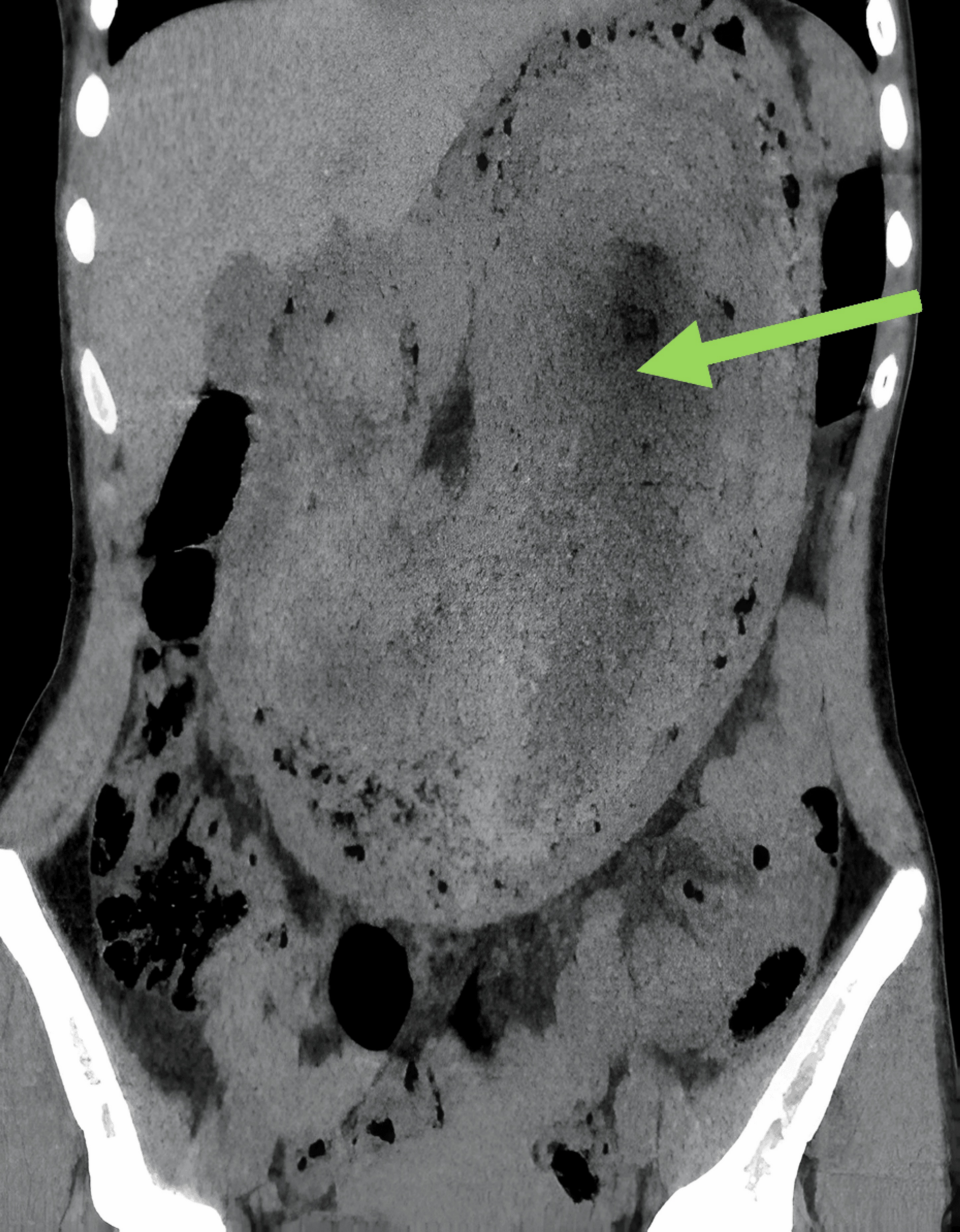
Abdomino-pelvic CT (coronal section) showing a diffuse, heterogeneous intragastric mass containing air and entrapped food debris (arrow).
